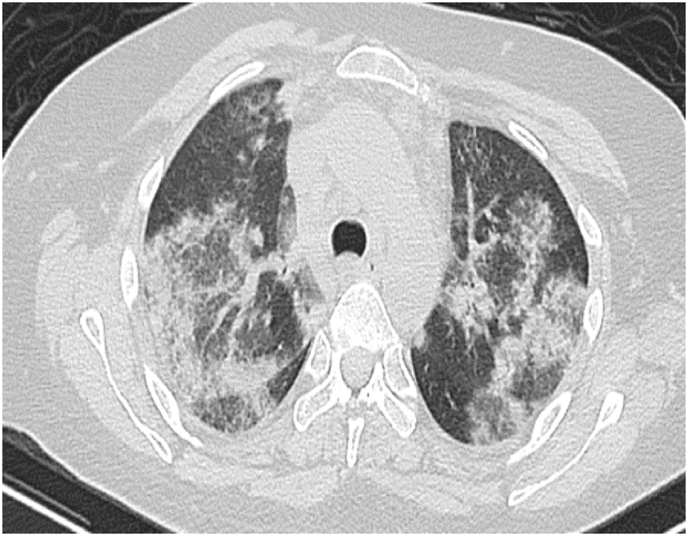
CT chest (lung window), January 2019: Bilateral ground-glass opacities with bilateral bronchial wall thickening of both upper lobes.
